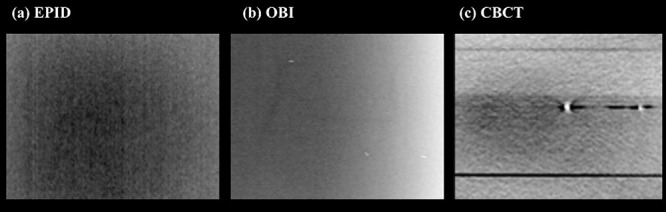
Images of our original titanium fiducial marker obtained by (a) electronic portal imaging device (EPID), (b) on-board imager (OBI), and (c) cone-beam CT (CBCT). The marker could be detected by OBI and CBCT, although it was not detected by EPID.
